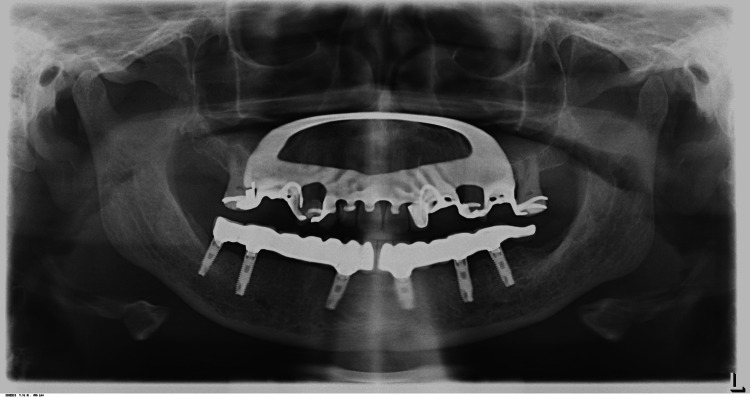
Eighteen-month follow-up panoramic radiograph
